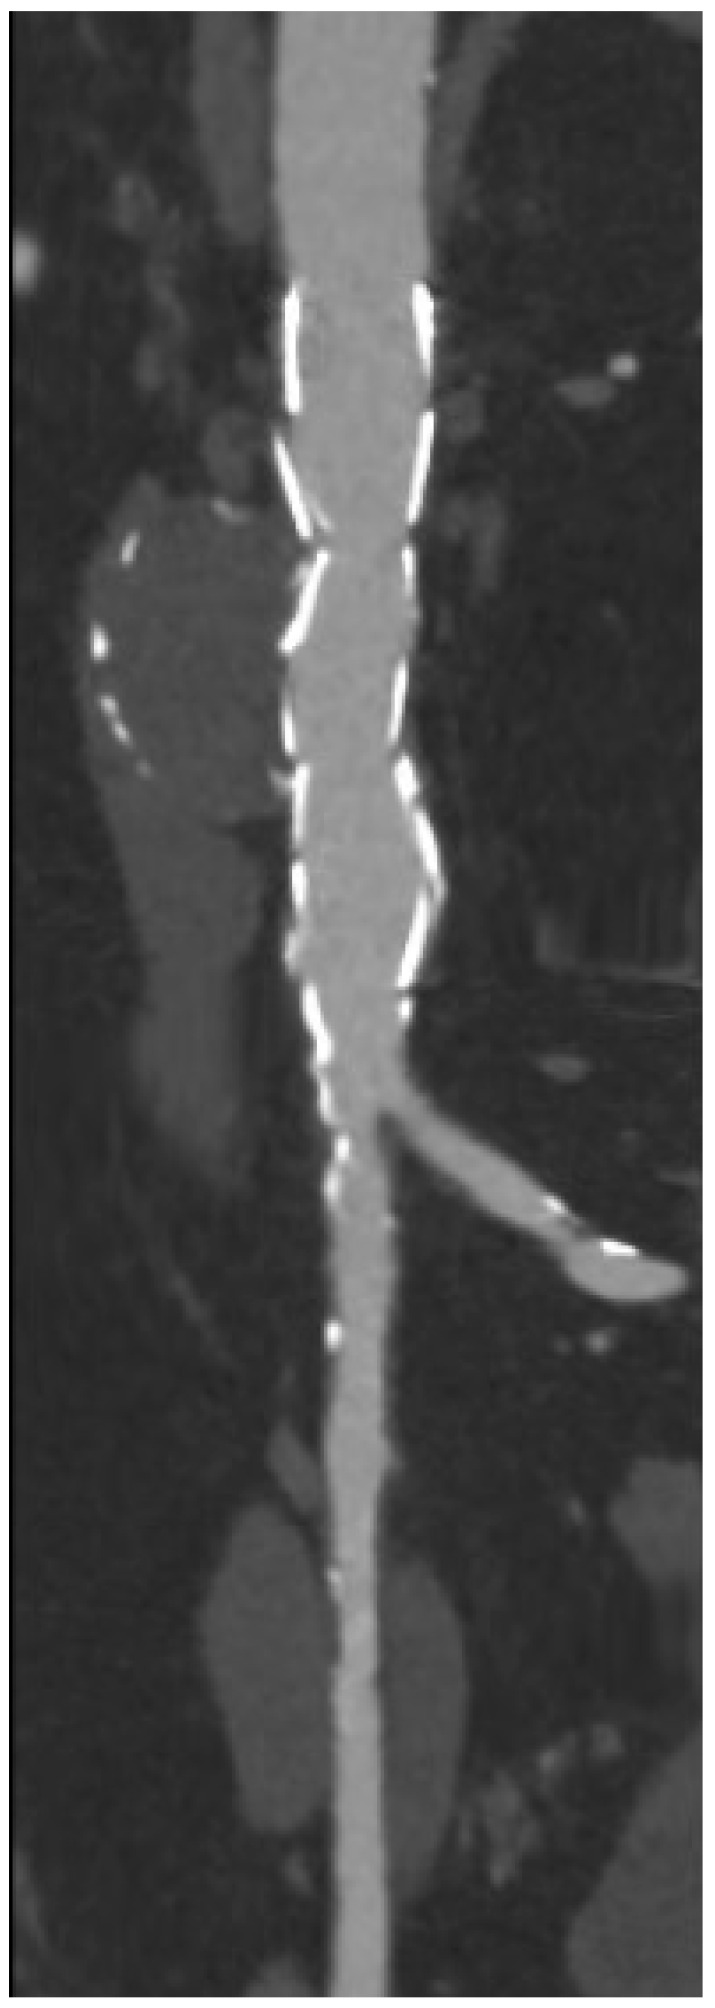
Same case. Follow-up CTA showing complete exclusion of the saccular aneurysm, optimal apposition of the endograft to the aortic walls, and preservation of both iliac axes.
